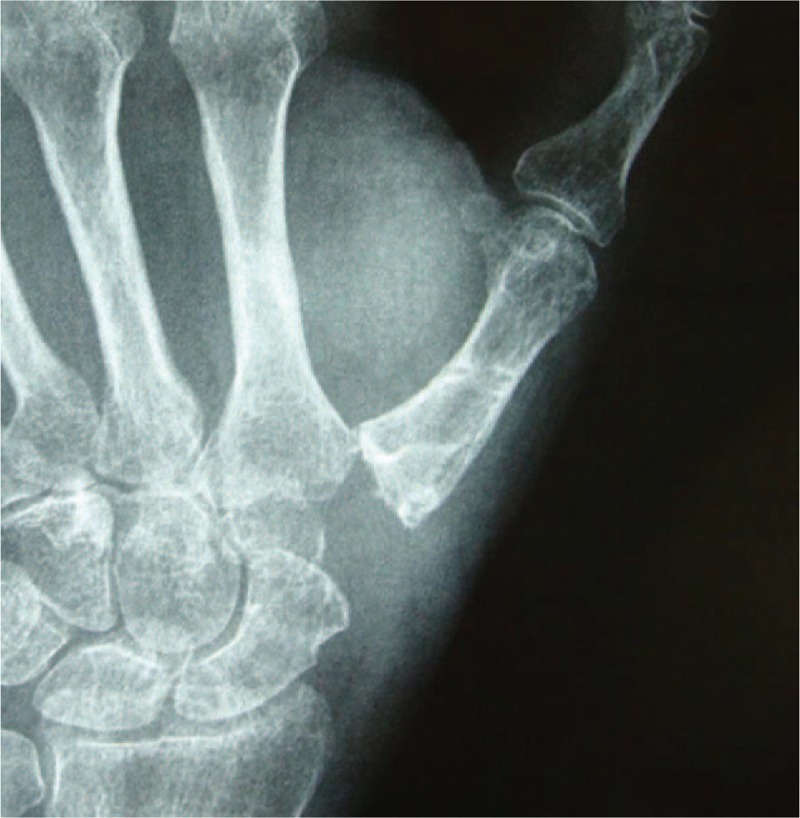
Hand x-ray showing the height of the trapezial space to be 9.8 mm.
